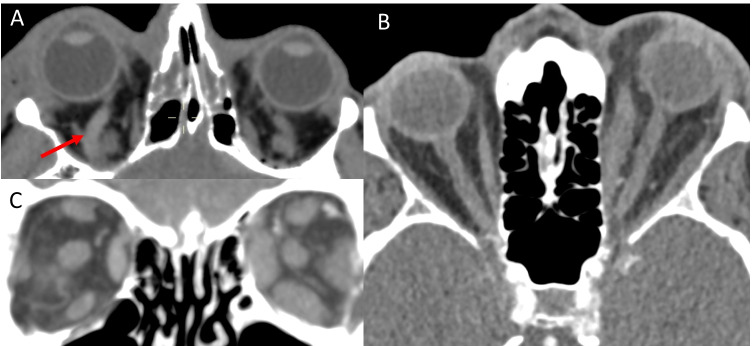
Carotid-cavernous fistula. Case 1—axial CT (A) of the orbits demonstrates enlargement of the right superior ophthalmic vein (arrow) and proptosis in a patient with a right direct carotid-cavernous fistula. Case 2—axial CT (B) showing left proptosis, enlargement of the left medial rectus muscle along with left globe tenting. Case 2—coronal CT (C) demonstrating enlargement of the left extraocular muscles
